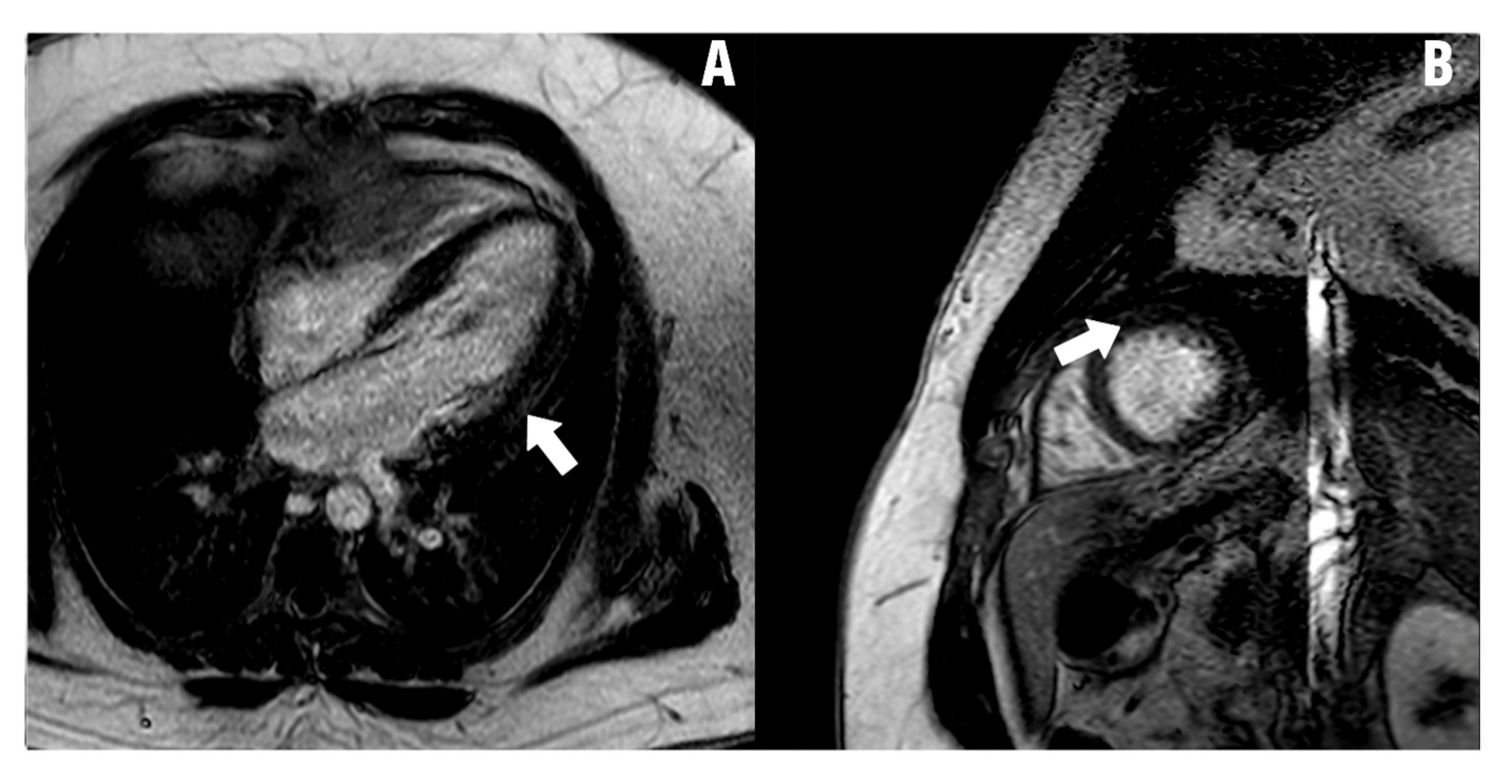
Cardiac MRI confirms acute myocarditis. Both arrows show acute myocarditis involving the left ventricle. According to the parametric mapping, myocardial native T1 time was 1022 ms and myocardial T2 time was 55 ms. (A) Horizontal long axis four chamber anterolateral wall view; (B) Short axis antero-apical wall view Image Credit: Mark Zenker
